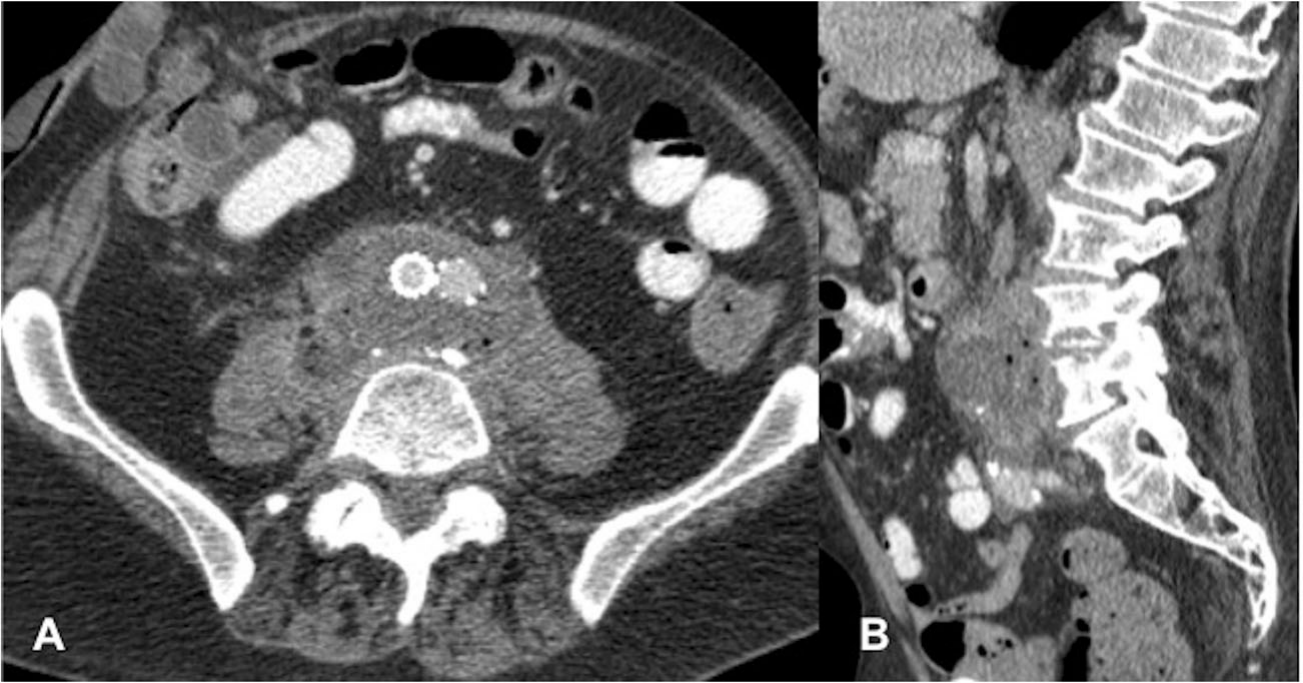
A 70-year-old man (patient 3). a Axial and b sagittal CT images 2 months post-EVAR. Note gas locules within the peripherally enhancing native aneurysm sac extending posteriorly to the vertebral column. There is a gas-containing right psoas/paravertebral abscess contiguous with the native aneurysm sac
